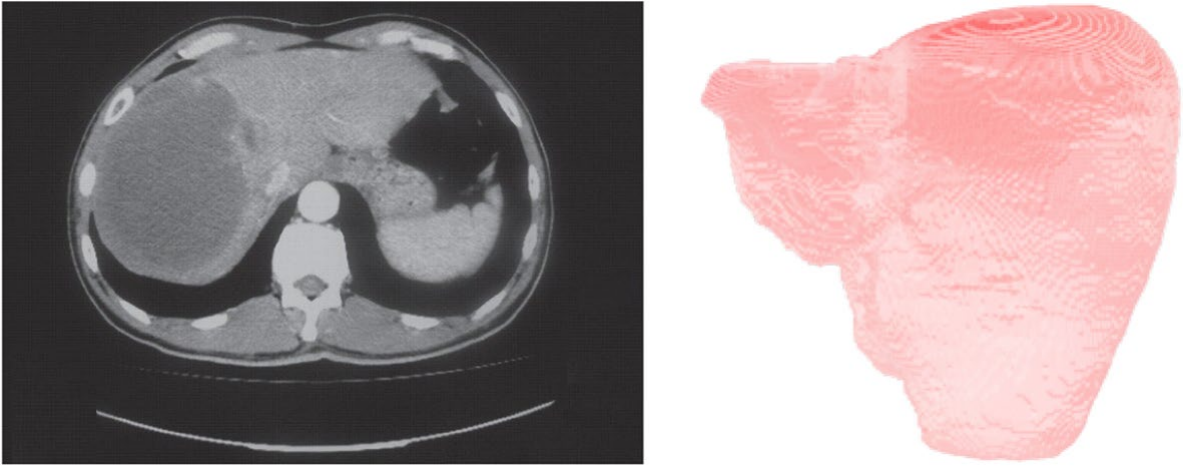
A slice from the input CT volume (left) and the 3D liver model (right)
